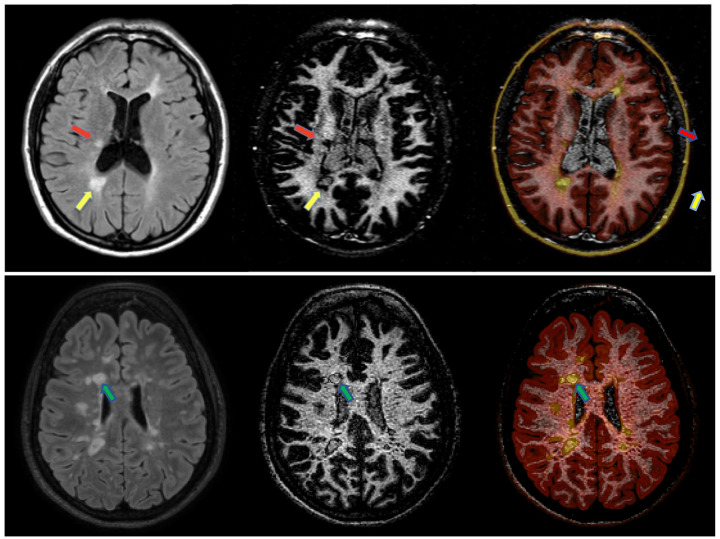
The first row describes the visualization, at 1.5 T, of a typical MS WM lesion on fluid attenuated inversion recovery (FLAIR) (left), characteristically surrounded by a dark rim (DRL) on a white-matter selective double inversion recovery sequence (WM-DIR) (middle, yellow arrow); a uniform hypointensity on WM-DIR (no-DRL) characterizing another WM lesion can be observed (red arrow). On the right an example of a color-coded FLAIR–WM-DIR fusion image to show where the “dark rim” is exactly located with respect to the FLAIR-visible contours. The second row shows a 3T example of DRL (green arrow) on WM-DIR (middle), FLAIR (left), and FLAIR-WM-DIR fusion (right).
